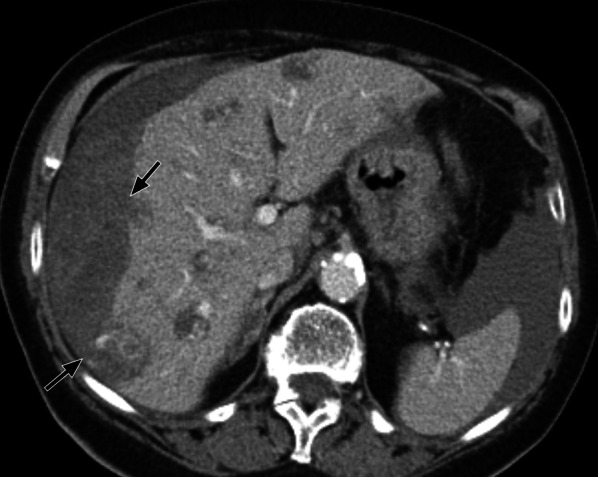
79-year-old woman with radiation-associated angiosarcoma of the breast. Axial contrast-enhanced CT shows multiple liver metastases which are of predominantly low attenuation with central and peripheral areas of enhancement. Haemoperitoneum is seen adjacent to the peripheral metastases (arrows)
